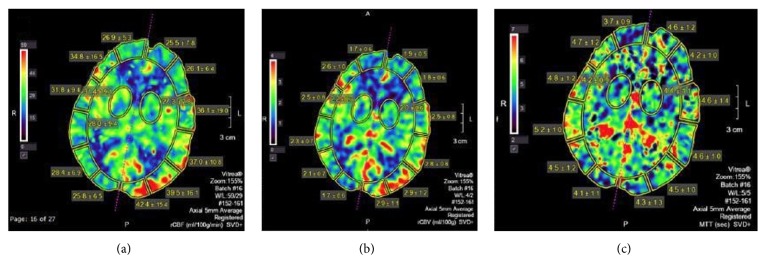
CT perfusion was performed 10 days after the initial MRI imaging. The postprocessed CT perfusion maps in axial plane at the level of the left parietooccipital cortical abnormality reveal increased cerebral blood flow (CBF) and cerebral blood volume (CBV) in the affected cortex on the left as compared to the normal brain parenchyma on the right. The time to peak (TTP) is decreased.
